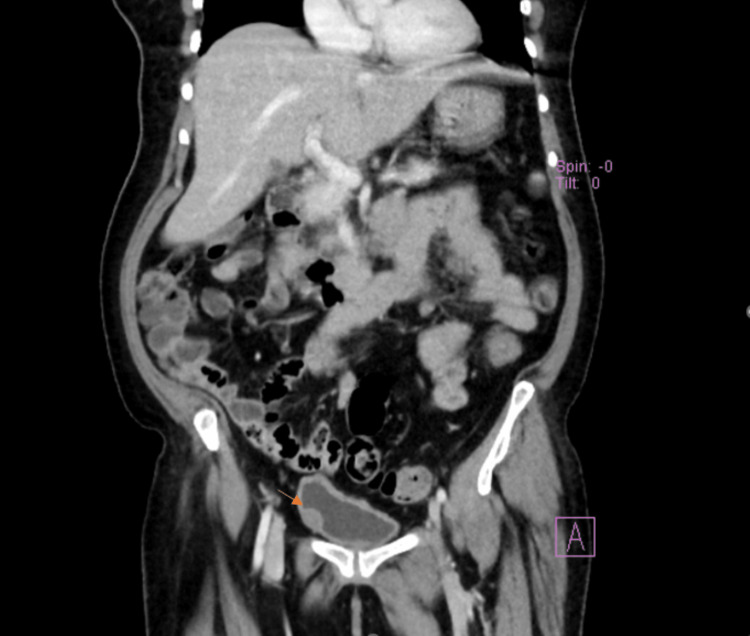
CT abdomen and pelvis with contrast. It shows a dome anterior diffuse urinary bladder wall thickening measuring 0.7 cm and focal right anterolateral wall elliptical shape soft tissue mass measuring 2 x 2 x 1.6 cm (arrow). Both kidneys are normal in size, shape and position with good parenchymal enhancement. There were no renal stones, hydronephrosis or focal lesions. Both ureters have normal lumen diameter, there is no filling defect. The liver, gall bladder, pancreas, and spleen are of normal size and density. No focal lesions. The right and left adrenal glands are bulky.
